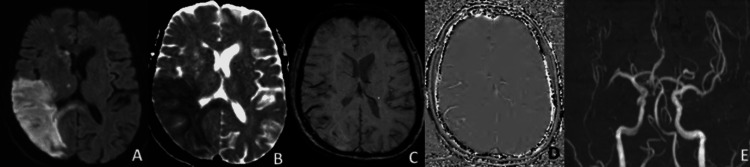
DWI (A), ADC (B), Magnitude (C), and phase (D) images of SWI sequence show infarction in left MCA territory with dark vessel sign in M3 and M4 branches of right MCA, which was confirmed by MR angiography (E).
